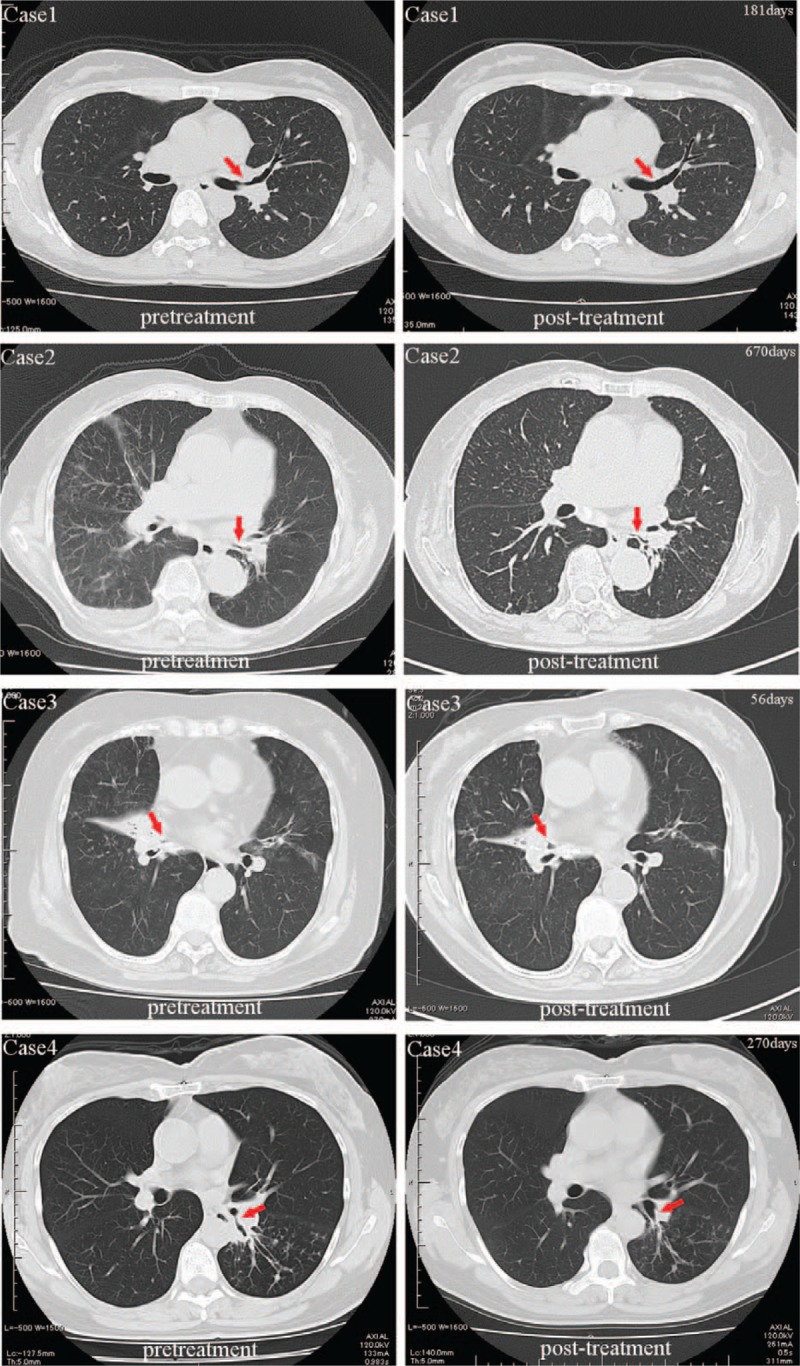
Chest computed tomography (CT) images before and after treatment in Cases 1–4. The figure in the right upper corner of each post-treatment CT image indicates the time (number of days) from the initiation of the administration an antituberculous drug. The arrows indicate the local steroid spray-applied airway regions. CT = computed tomography.
